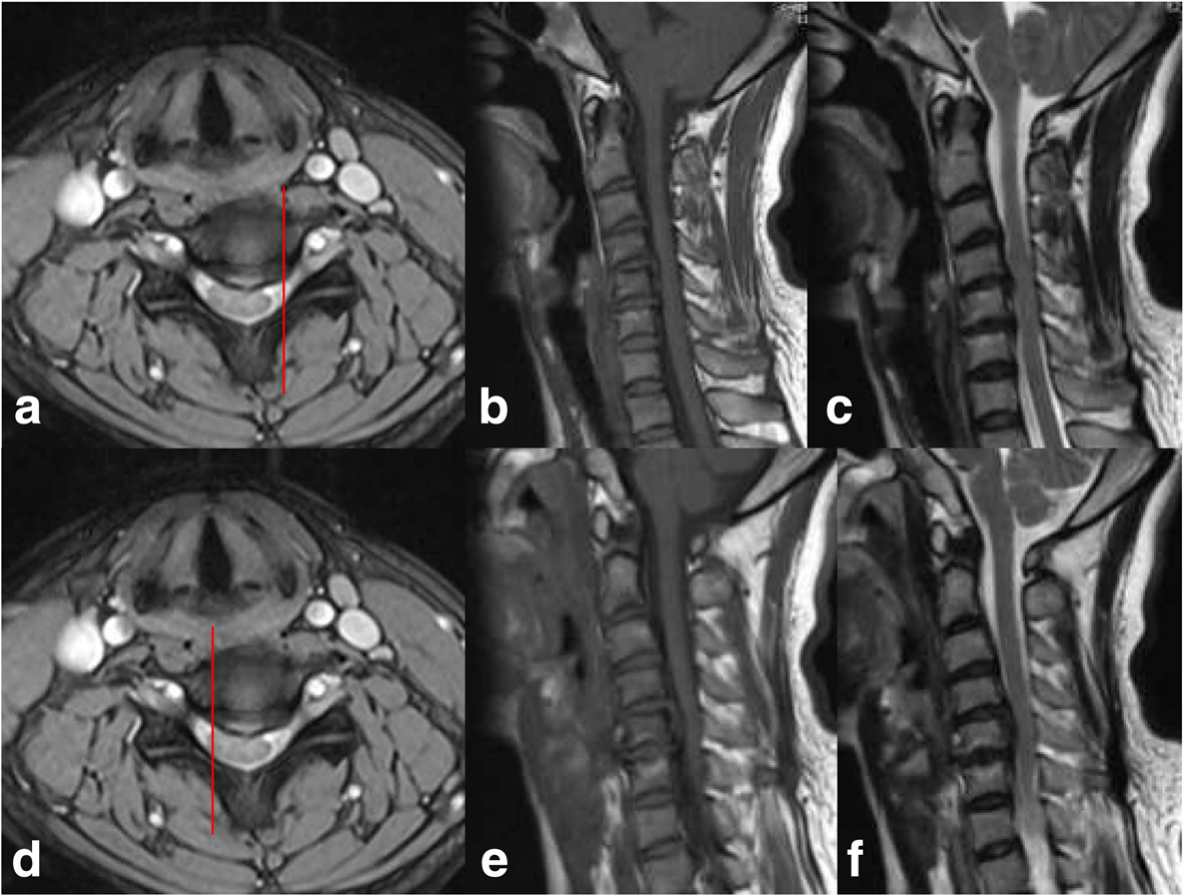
Conventional Modic changes. a, d The axial view of MRI and the red lines were the location of b and c in a and e and f in d. Whether on the left side of on the right side, T1 and T2 hyperintense always existed, which indicated the signal changes throughout the whole endplant
